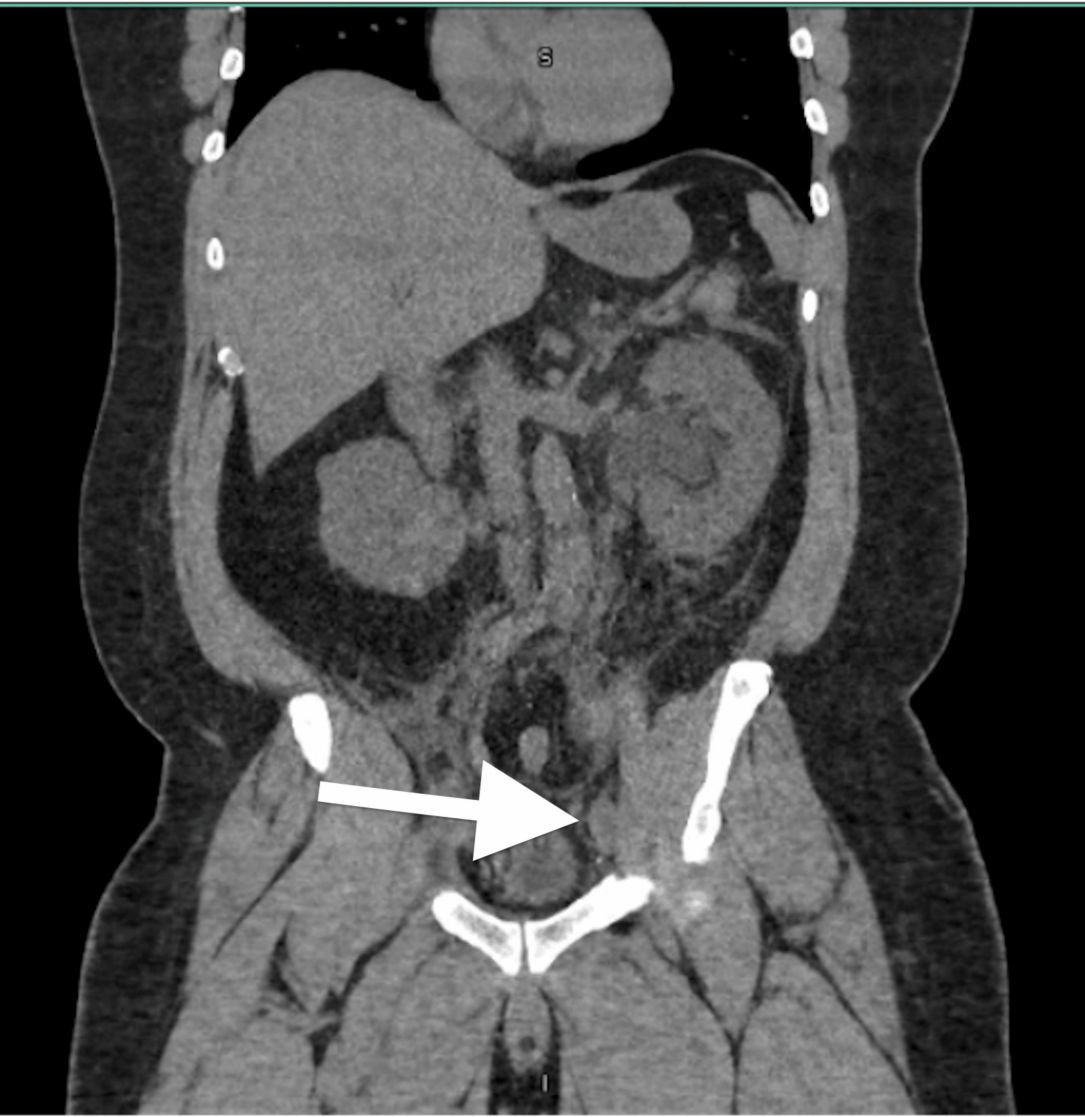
Left-sided hydroureteronephrosis on coronal non-contrast CT of the abdomen on postoperative day two.
